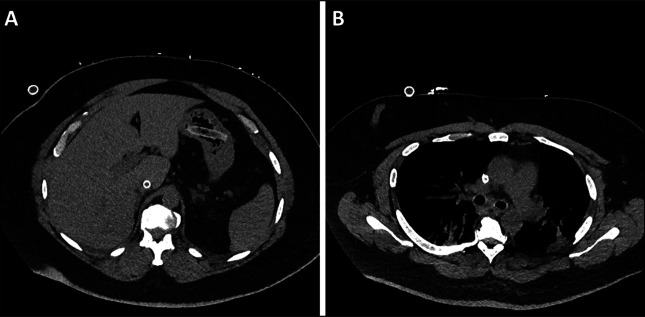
Abdominal CT without contrast shows the presence of a stent in the stomach (A); chest CT without contrast shows an absence of a stent (B). CT, contrast tomography.
